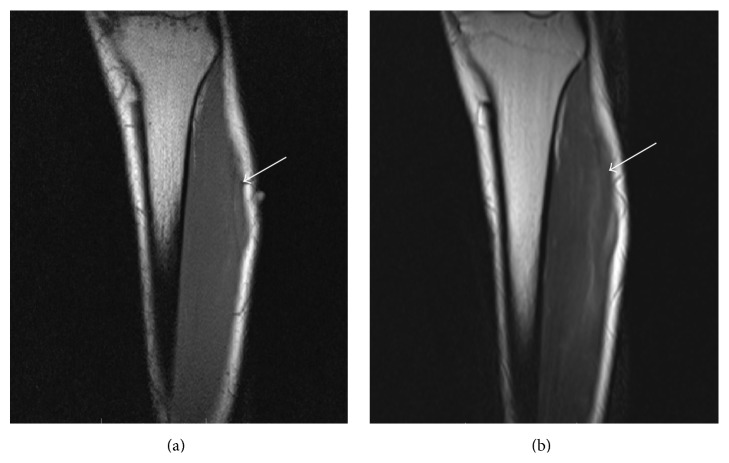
(a) Coronal section of left leg T1-weighted sequence showing defect at the level of the marker (marked by arrow) with outward bulging of underlying tibialis anterior through the defect. (b) Coronal section of left leg T2-weighted sequence outward bulging of tibialis anterior due to muscle herniation through the defect (marked by arrow).
